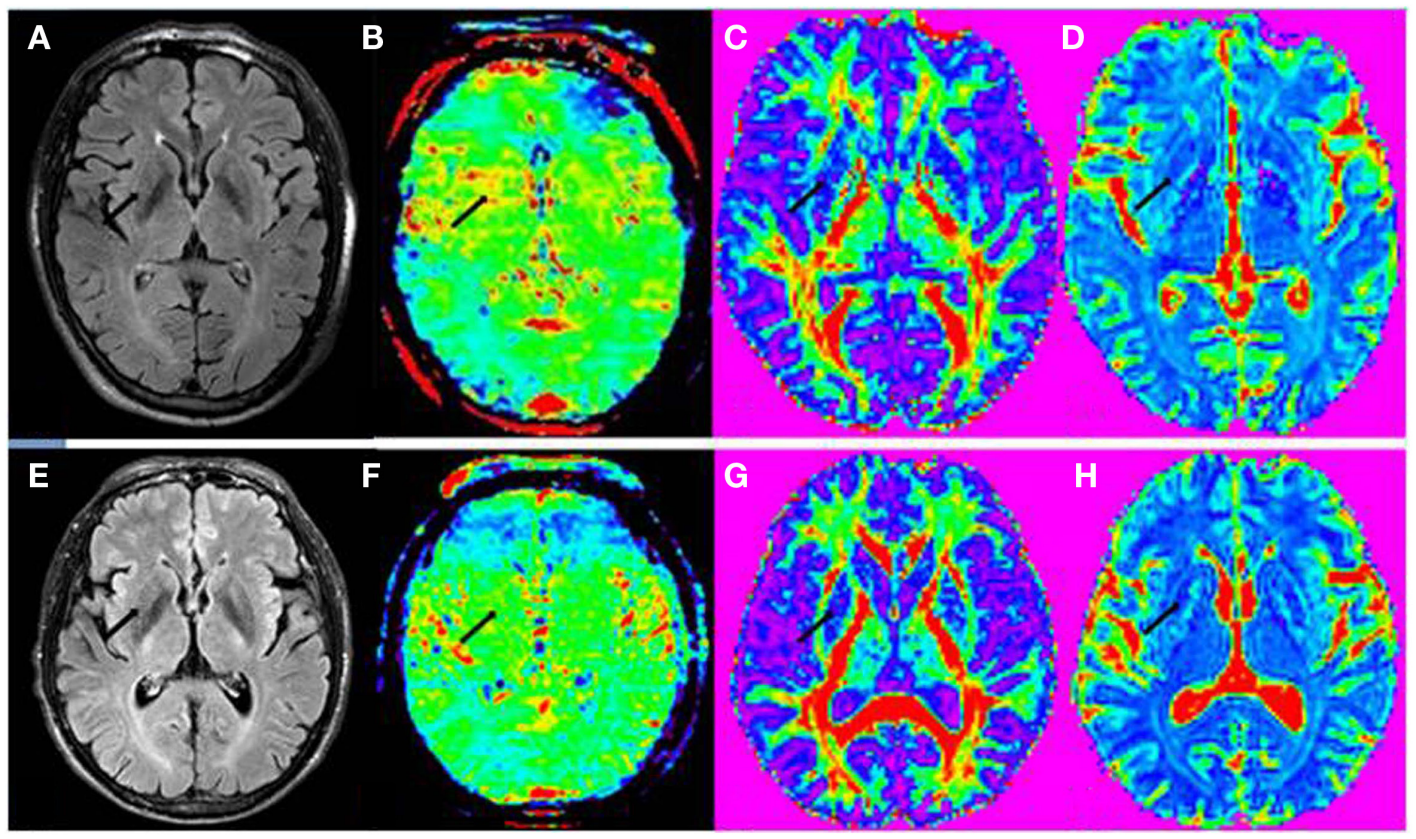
(A) FLAIR image, (B) APT-weighted image, (C) FA image, and (D) MD image of a PD patient (male; 74 years old; H&Y stage 2.5). (E) FLAIR image, (F) APT-weighted image, (G) FA image, and (H) MD image of a normal control (male; 61 years old). The CEST/APT imaging acquisition protocol provided B_0_ inhomogeneity-corrected, APT-weighted images with sufficient signal-to-noise ratios. The APT-weighted intensities in regions of the striatum (black arrow) were higher in PD patient than in normal control. The FA and MD values in regions of the striatum (black arrow) seemed to be similar for this PD patient and normal control.
